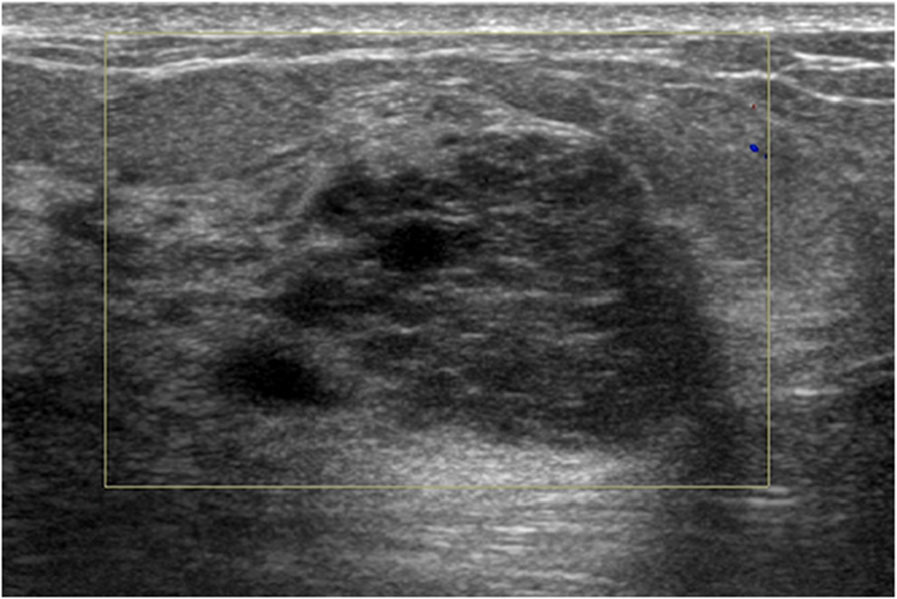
Ultrasonographic findings of a MUMPC in a 41-year-old patient with a palpable left breast tumor. US shows a 3.0 × 2.2 × 1.9 cm parallel, irregular, and non-circumscribed tumor with mixed solid and cystic components and posterior acoustic accentuation (BI-RADS 4B). No signal of blood flow was found in the mass
